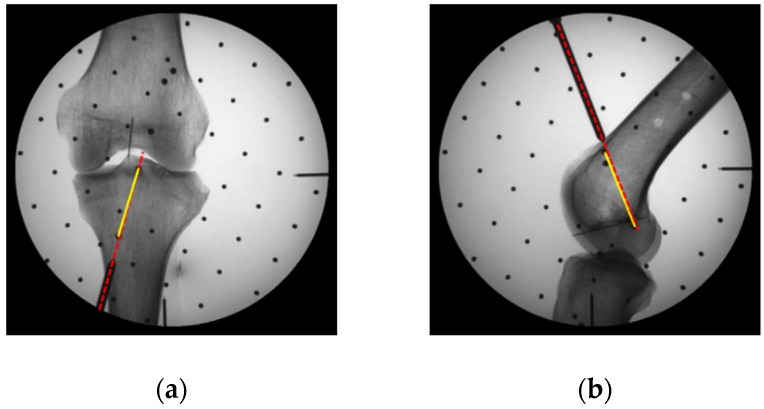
X-Ray images of positioning of the tunnels, planning tunnels through 2 steel balls was marked in yellow line and positioning tunnel was marked in red line: (a) Tibia planning tunnel; (b) Femoral planning tunnel.
